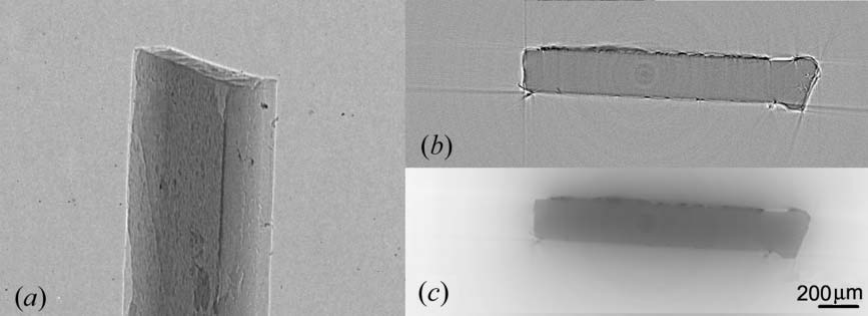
(*a*) X-ray projection of a plastic foil, (*b*) reconstructed CT slice using *Octopus* and (*c*) reconstructed CT slice using *X-TRACT*.
